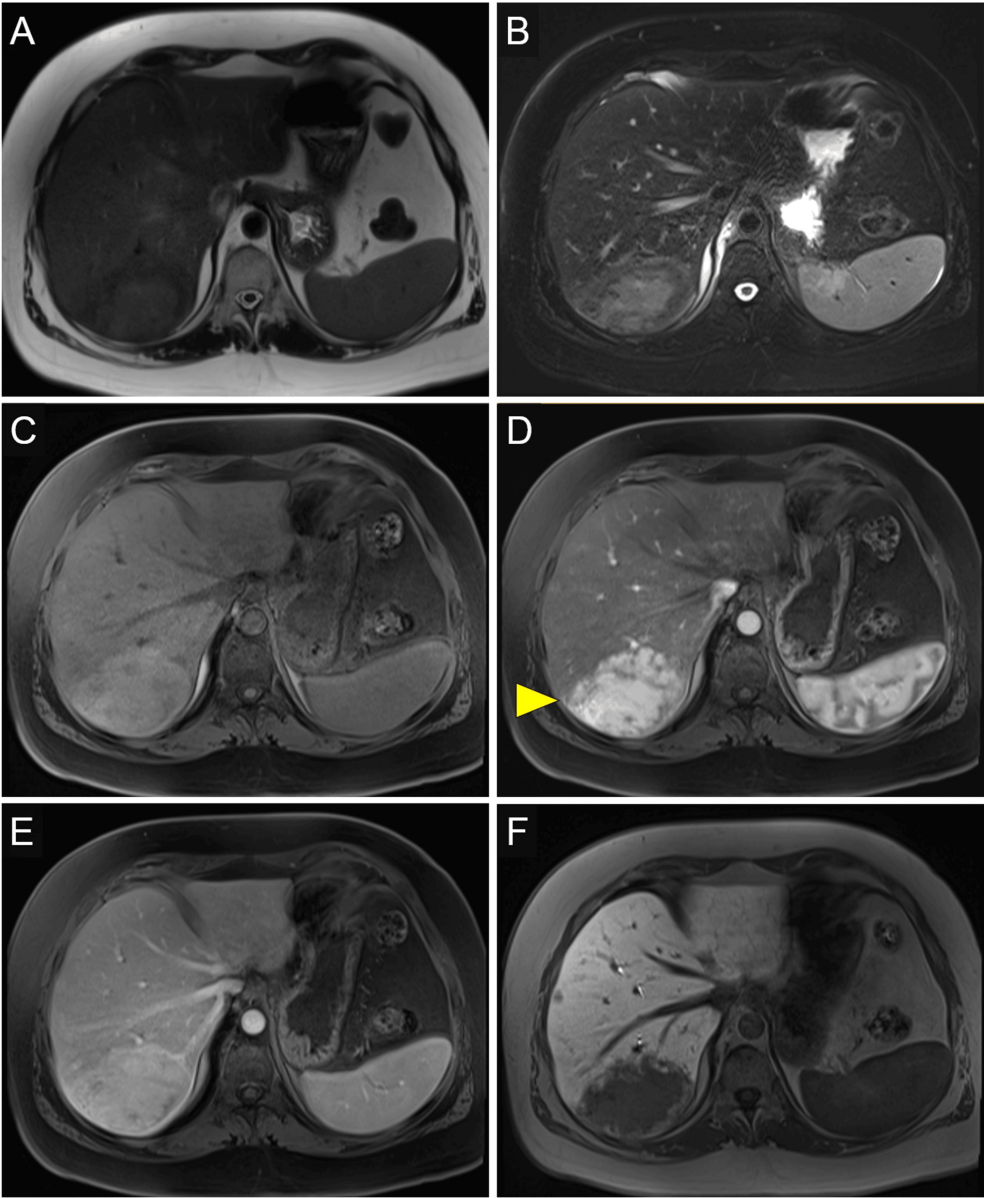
Hepatic adenoma in segment VI/VII Hepatic adenoma in segment VI/VII measuring 9.0 x 5.8 cm (arrowhead). MRI sequences include T2-weighted (A), T2-weighted with fat saturation (B), and T1-weighted images with fat saturation obtained over multiple phases, including pre-contrast (C), post-contrast arterial phase (D), venous phase (E), and hepatobiliary phase (F).
